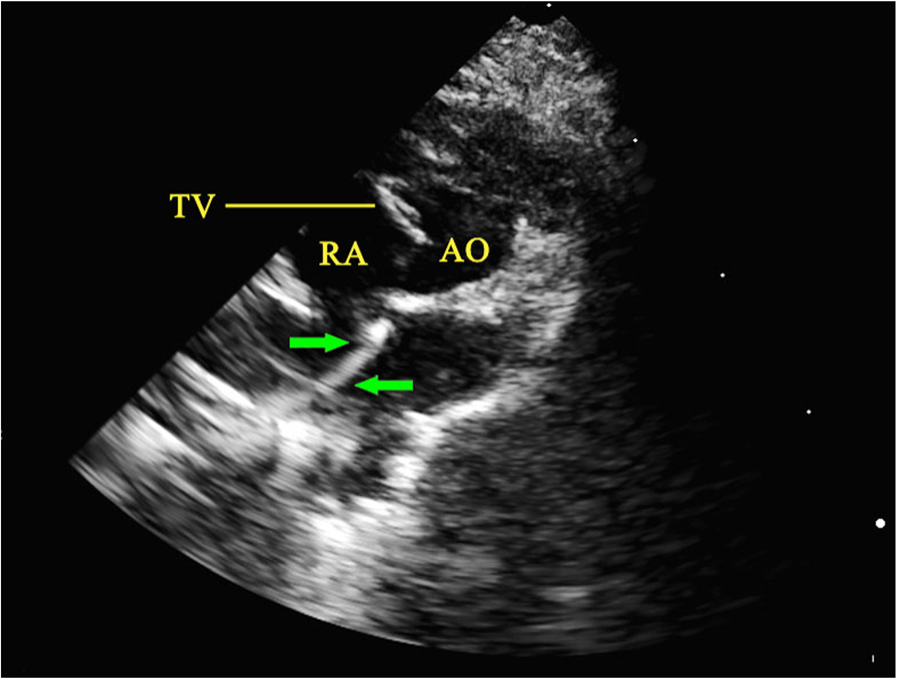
Entry of the catheter system into the right atrium as visualized by echocardiography. The catheter system was visualized entering the right atrium, close to the atrial septum in aortic short axis view by echocardiography. The green arrows indicate the position of the catheter system. AO, aorta; RA, right atrium; TV, tricuspid valve.
